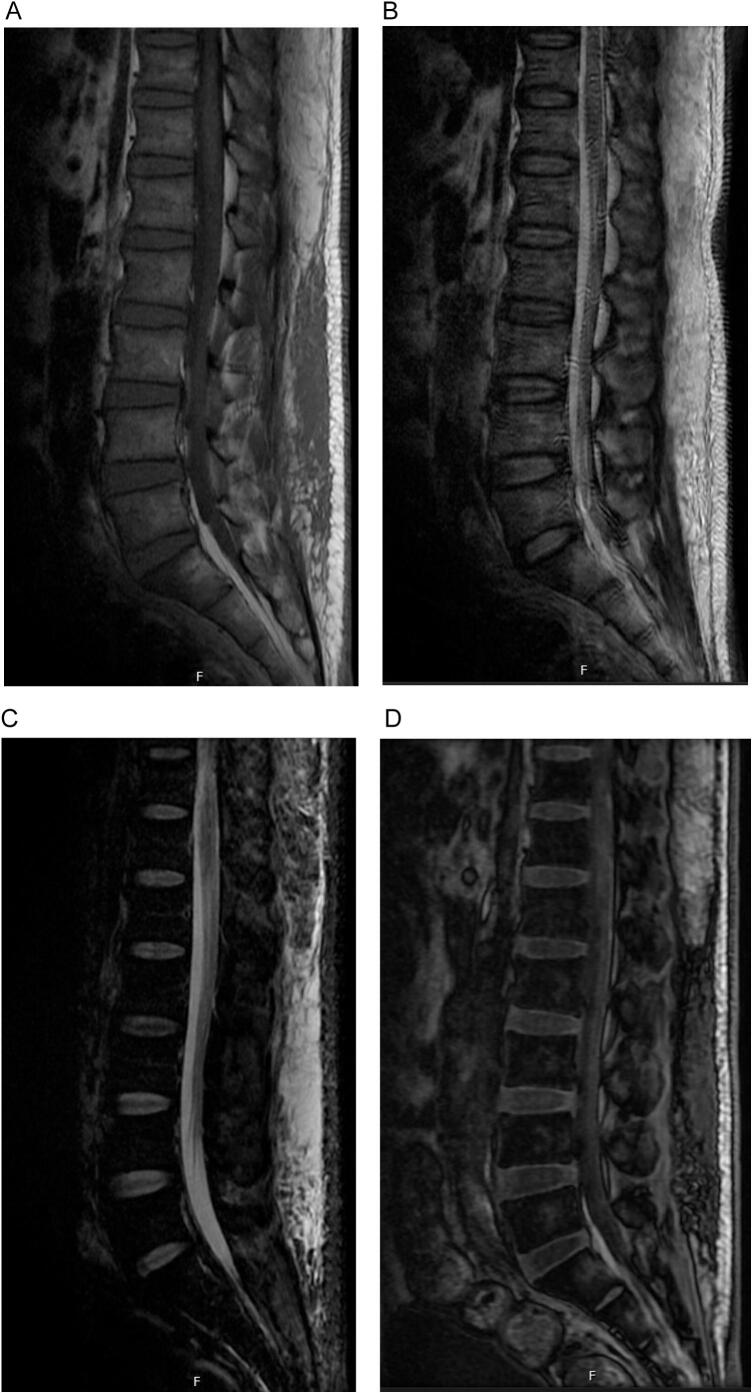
Figure 2: MRI spine. 2A: Sagittal T1 graded with no evidence of disc prolapse. 2B: Sagittal T2 graded with no evidence of local compression on the cord, disc prolapse or subluxation. 2C: Sagittal STIR (short Tau inversion recovery). 2D: Sagittal 3dDualEcho.
